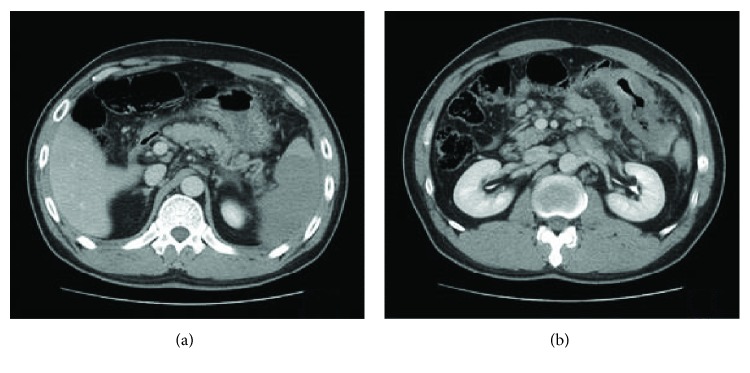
CT scan of patient no. 5. (a) Axial section of the CT scan showed an infarction of the lower pole of the spleen on admission. (b) Axial section of the CT scan showed a colon tumor of the splenic flexure.
